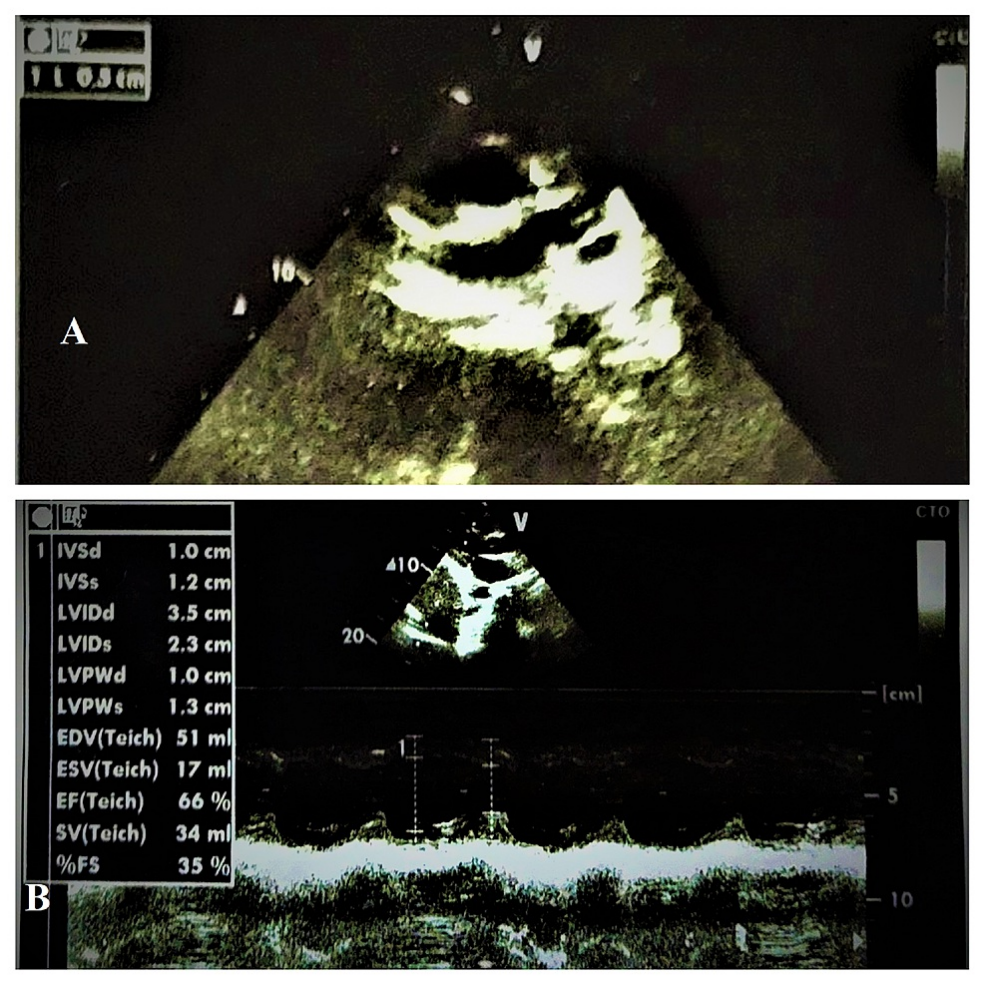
Echocardiograph of the patient showing dilated coronary artery (left main coronary artery (LMCA): 5 mm) (A) and good left ventricular systolic functions (ejection fraction: 66%) (B).
